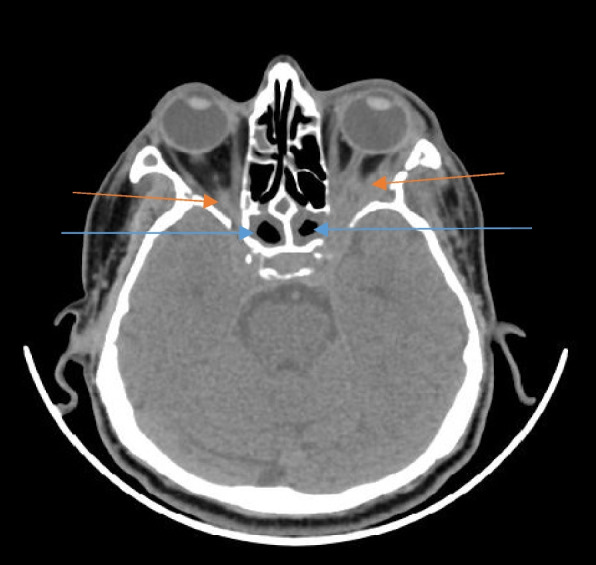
Axial section of a plain CT of the brain in a patient with rhino-orbital mucormycosis showing mucosal thickening in bilateral sphenoid sinuses (blue arrows) with spread into the intra-conal spaces of bilateral orbits as seen by involvement of intra-orbital segments of bilateral optic nerves with surrounding soft tissue and fat stranding (red arrows)
